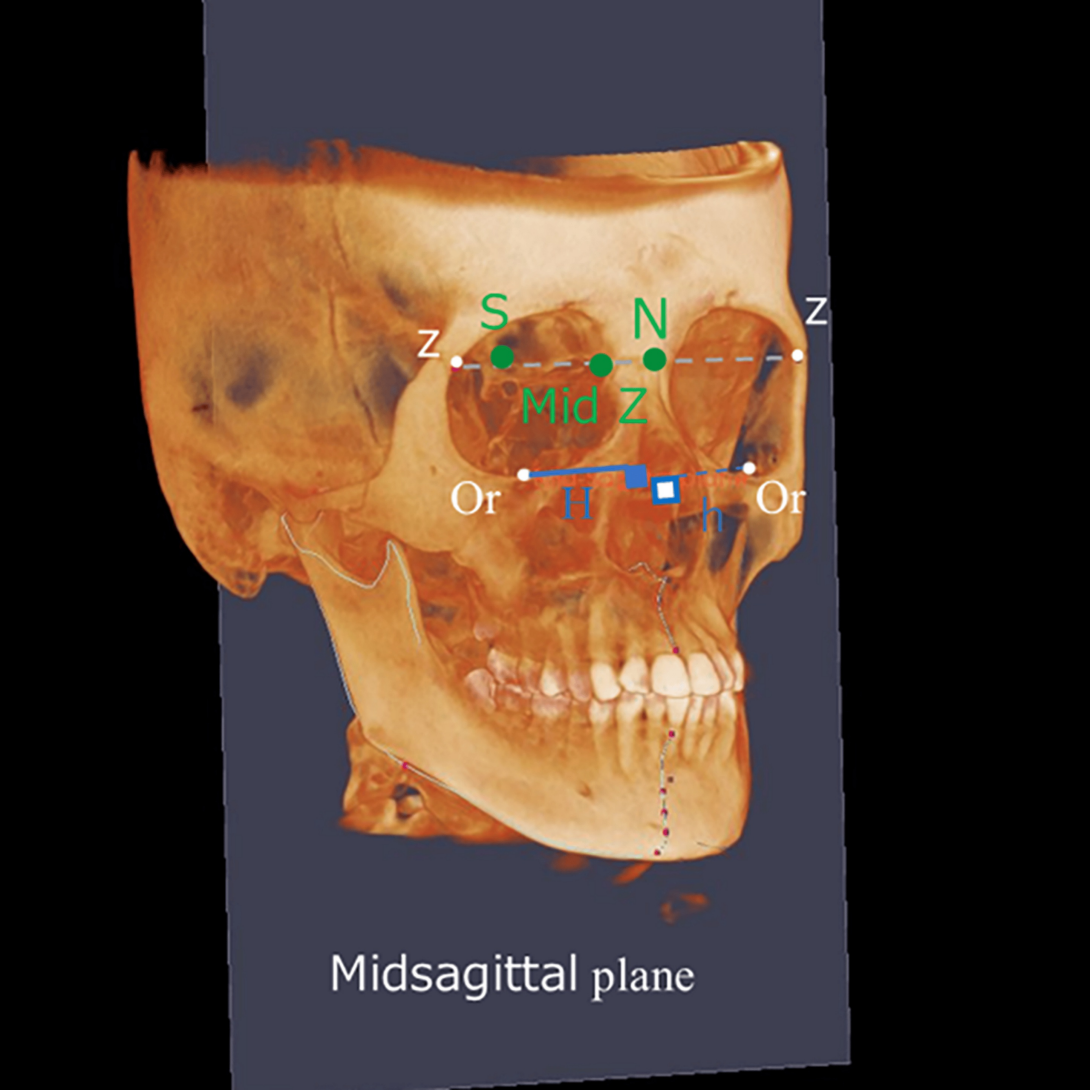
The midsagittal plane composed of N, S, and Mid Z, along with the H-line using cone-beam computed tomography images. H: perpendicular line from the right Or to the midsagittal plane; h: perpendicular line from the left Or to the midsagittal plane
